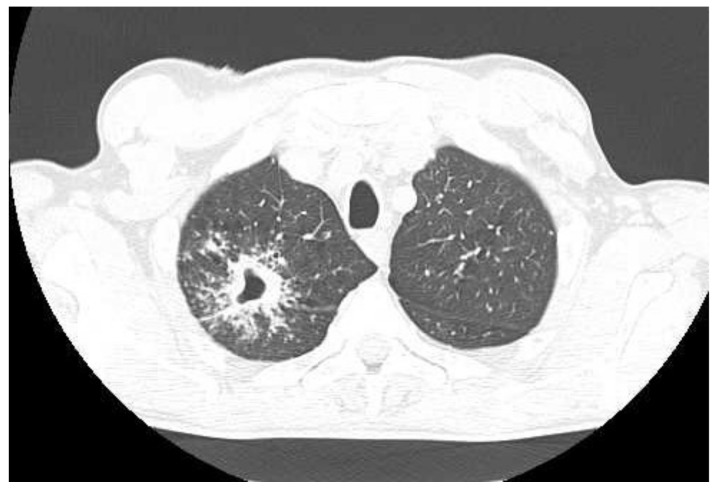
CT scan of the chest taken at the emergency department on admission to hospital in May 2021.
